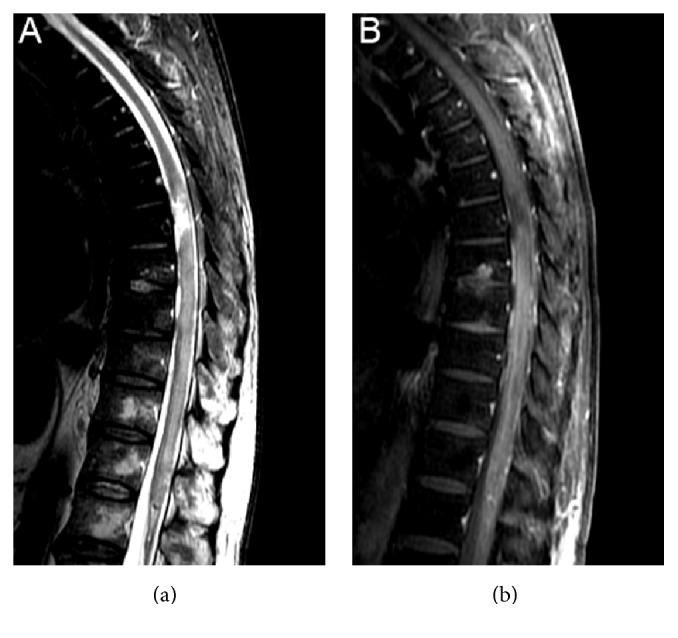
Spinal cord magnetic resonance imaging. Sagittal T2-weighted MRI shows a high signal intensity lesion on T6-T7 levels and a longitudinally extensive tumefactive hyperintense lesion from T6 to the medullary cone (a) with enhancing on postcontrast series (b).
